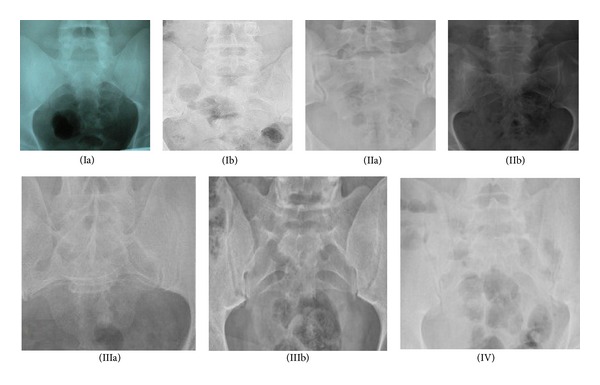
Types of the Castellvi radiographic classification system. (Ia) Case number 1746. (Ib) Case number 312. (IIa) Case number 1021. (IIb) Case number 1290. (IIIa) Case number 1075. (IIIb) Case number 1330. (IV) Case number 1988.
